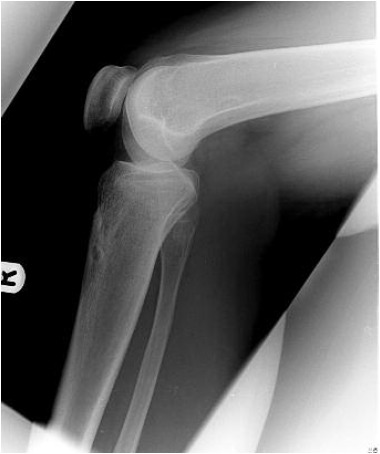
Lateral Xray of the knee taken on
